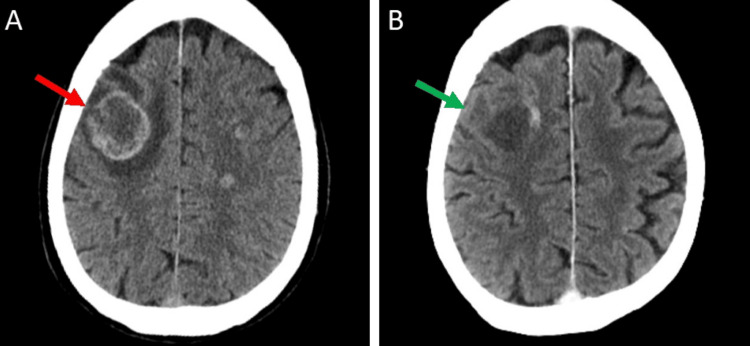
CT brain with IV contrast before and after whole brain radiation. (A) Initial CT brain with IV contrast showing a large parenchymal lesion at the right frontal lobe (red arrow). (B) Follow-up CT brain with contrast four weeks after whole brain radiation, showing reduction in size and edema of the same lesion (green arrow). CT: computed tomography.
